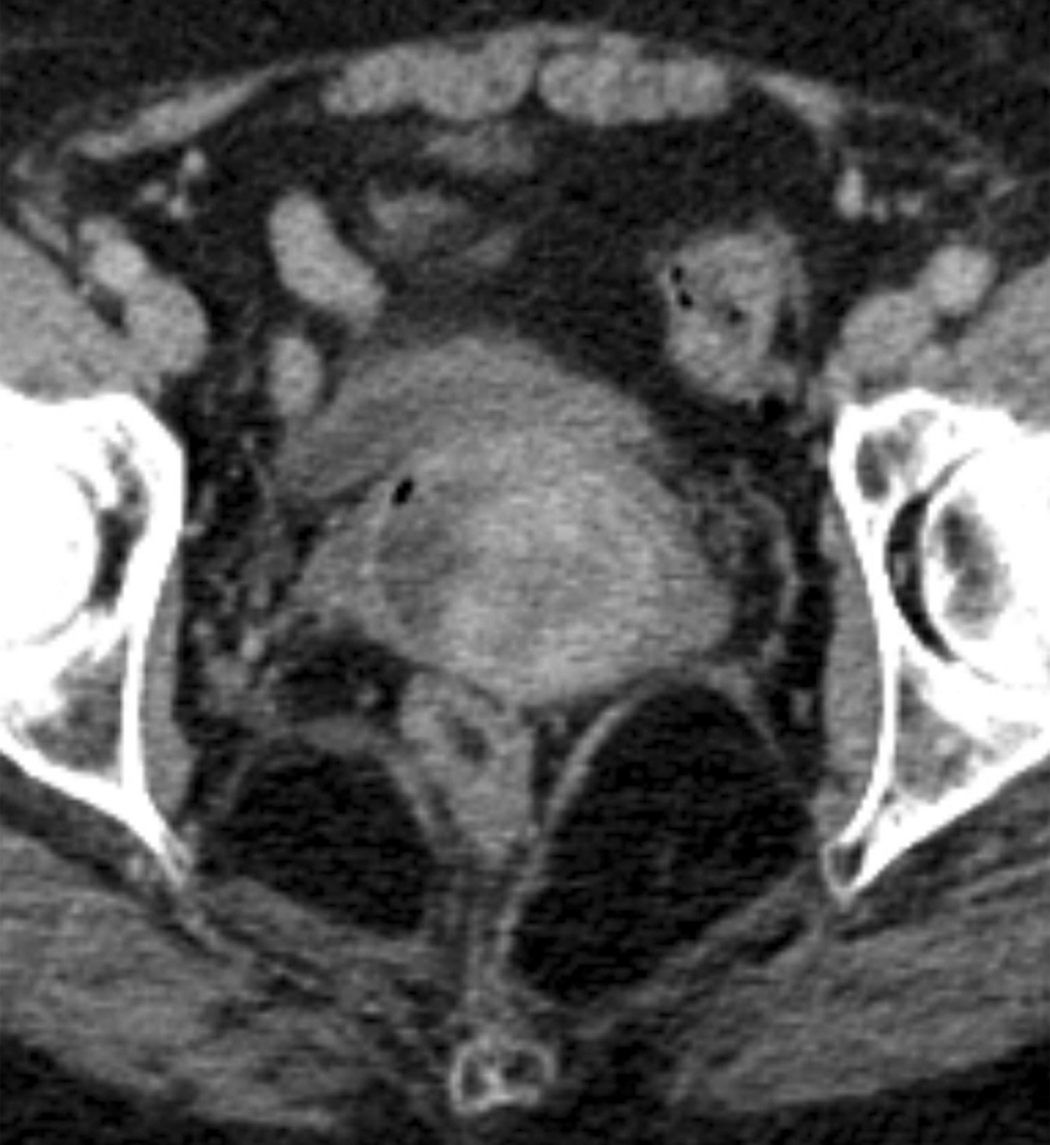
Axial portal venous phase contrast-enhanced CT image of the upper vagina demonstrating a mixed attenuation mass with patchy avid contrast enhancement.
